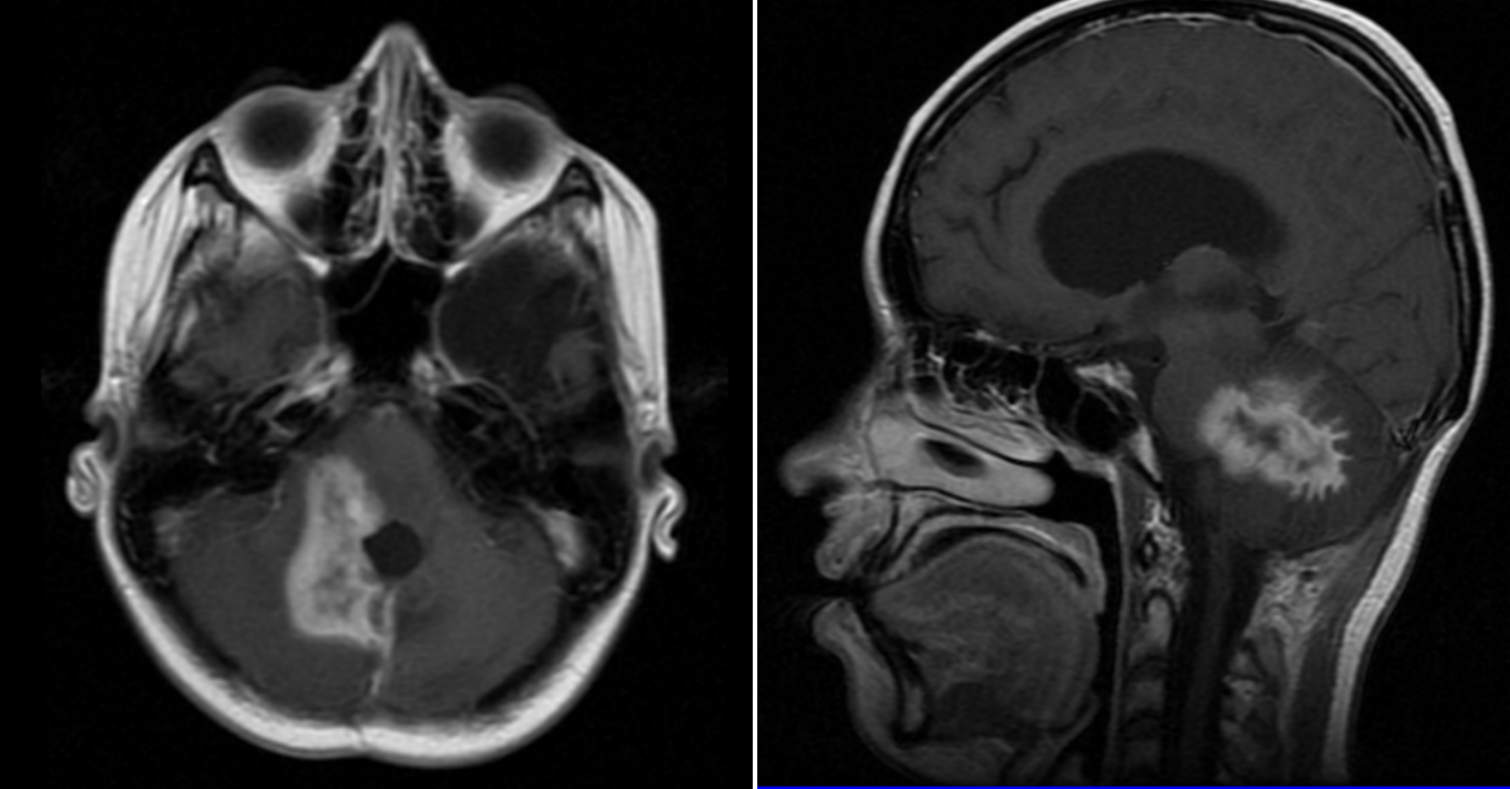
Magnetic resonance imaging (MRI) showing cerebellar vermis progression in November 2012 Left: Axial view Right: Sagittal view
